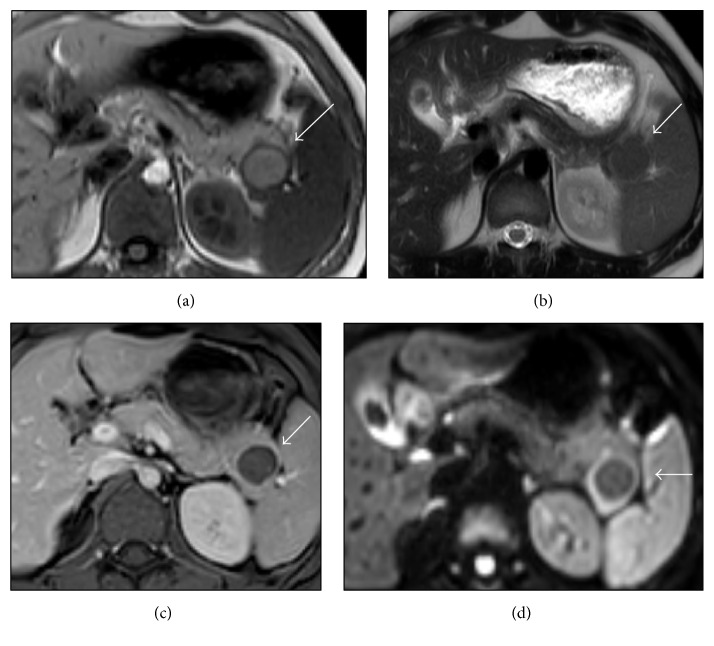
MRI exam showing (a) T1-weighted image, (b) T2-weighted image, (c) Gd-enhanced image, and (d) DWI image of the lesion. Note how DWI best demarcates soft-tissue rim surrounding cystic component, with equivalent signal intensity to spleen.
